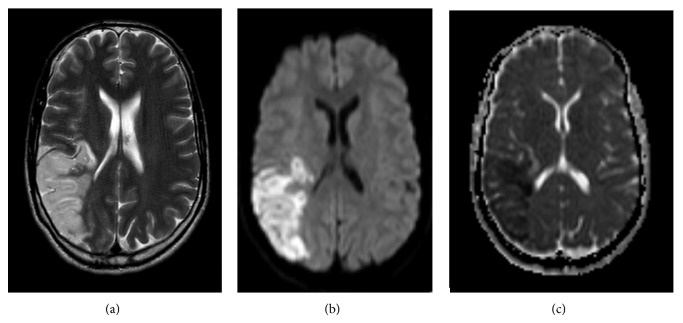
MRI of the brain. (a) T2-weighted image, (b) diffusion weighted image, and (c) apparent diffusion coefficient (ADC) map, showing high signal intensity on the T2-weighted and diffusion weighted image and low signal intensity on the ADC map in the vascular territory of the posterior division of the right middle cerebral artery, consistent with recent ischemia.
